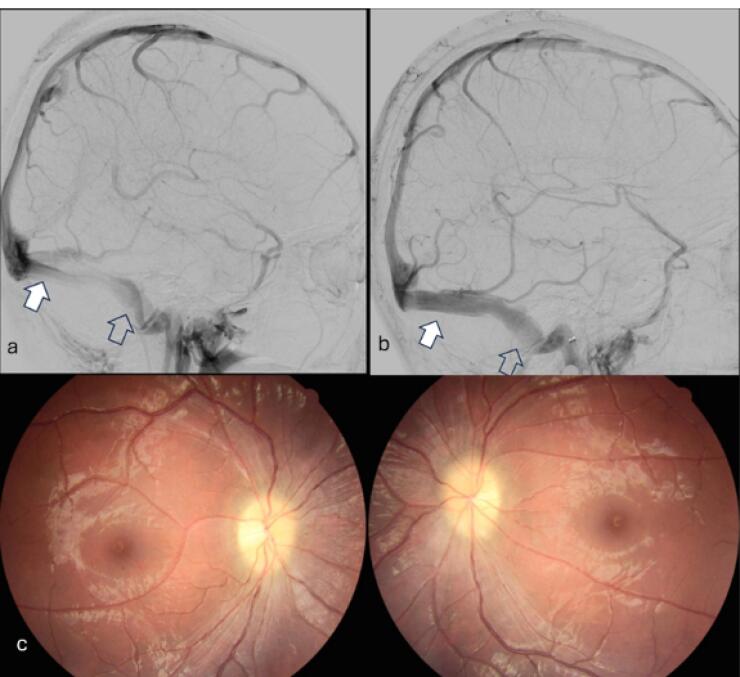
Idiopathic intracranial hypertension (IIH) often presents with symptoms such as headache (75‒94%), pulsatile tinnitus (52%‒60%), photophobia (42%‒73%), and visual loss or blurring (32%).^1,2^ According to the revised diagnostic criteria,^3^ IIH is diagnosed by meeting criteria A to E in the absence of known secondary causes. A ‘probable’ diagnosis of IIH may be considered if only criteria A–D are met. These criteria are defined as follows: (A) Papilledema, (B) Normal neurological examination except for cranial nerve abnormalities, (C) Neuroimaging showing no hydrocephalus, mass, structural lesion, abnormal meningeal enhancement, or venous sinus thrombosis, (D) Normal CSF composition, and (E) Raised lumbar puncture opening pressure (>25 cmH_2_O in lateral decubitus). Additionally, a diagnosis of IIH can be made in patients without papilledema if criteria B–E are satisfied and the patient has a unilateral or bilateral abducens nerve palsy. The diagnosis of IIH can be also ‘suggested’ based on at least three of the following neuroimaging criteria which indicate signs of elevated intracranial pressure: (*i*) empty sella, (*ii*) flattening of the posterior aspect of the globe, (*iii*) distention of the perioptic subarachnoid space with or without a tortuous optic nerve, and (*iv*) transverse venous sinus stenosis, in patients who meet criteria B–E but do not have papilledema or sixth nerve palsy. However, the diagnostic value of abducens nerve palsy as a separate diagnostic criterion has been questioned by Korsbæk et al.^4^ Since empty sella can also occur in healthy individuals and is associated with obesity and other conditions, Beier et al suggest that an empty sella may not be directly associated with IIH. Instead, they propose that the presence of 2 of the 3 MRI signs (posterior globe flattening, optic nerve disc protrusion, and transverse sinus venous stenosis) are the key features for diagnosing IIH.^5^
